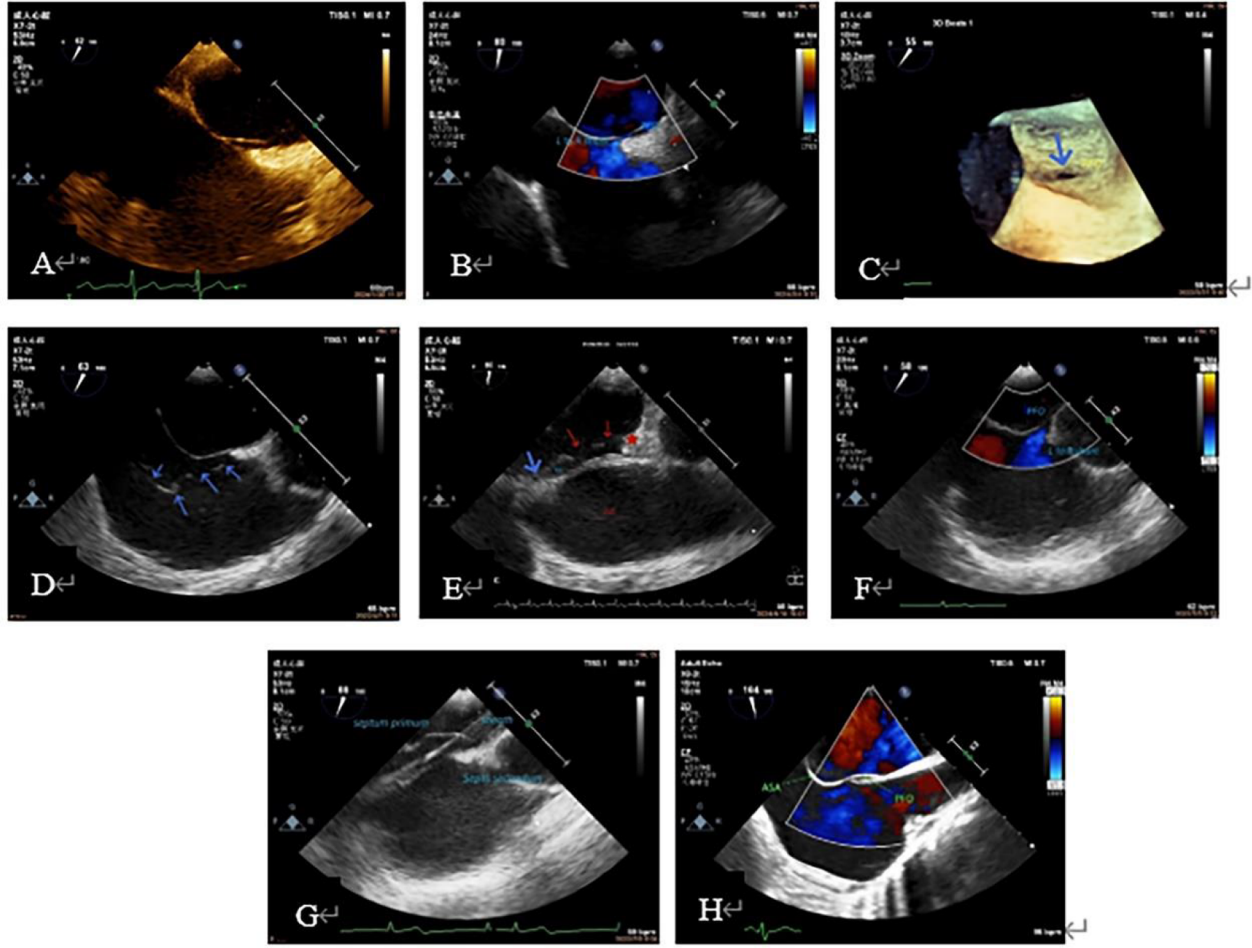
Preoperative TEE to understand PFO shape, size, shunt, thickness and mobility of the primary septum, whether it is combined with ASA, excessive eustachian valve, and whether it is combined with other structural problems that need to be treated. (A): PFO tunnel showed a small RA opening and a large LA opening. (B): LRS of PFO; (C): PFO under 3D ultrasound (blue arrow); (D): excessive Eustachian valve; (E): the primary septum of the PFO (shown by blue arrow) was connected with the coumadin ridge (shown by red star). The PFO was continuously open, and two continuous interruptions were observed on the primary septum (shown by red arrow). (F): Preoperative diagnosis of ASD, intraoperative TEE showed a large PFO; (G): primary septum with large mobility. (H): TEE showe ASA. 3D, three-dimensional; ASA, atrial septal aneurysm; ASD, atrial septal defect; LA, left atrial; LRS, left-to-right shunt; PFO, patent foramen ovale; RA, right atrial; TEE, transesophageal echocardiography.
